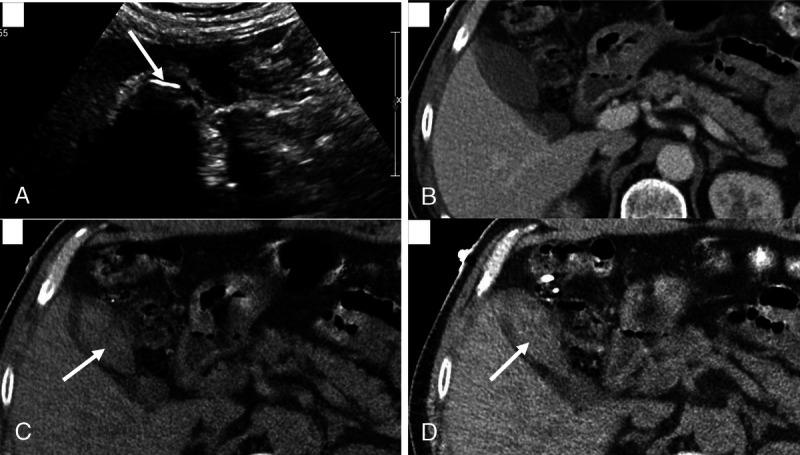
Ultrasound showing gallbladder filled with a large stone (A), which was not seen in the PC image (B), however evident on both VNC (C) and 50-keV VMC (D) images.
